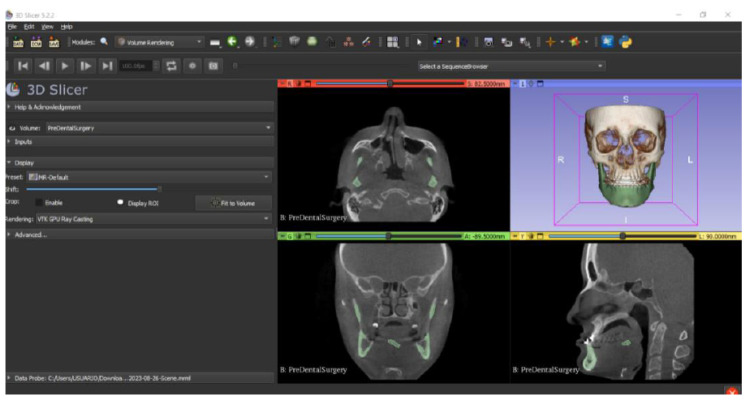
DICOM CBCTDentalSurgery Sample Data PreDentalSurgery in “Four-Up” configuration.
